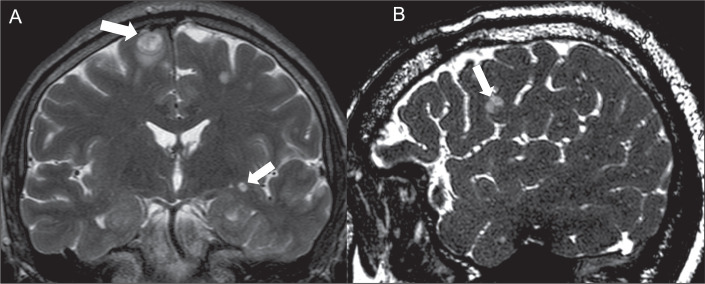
MRI scans of a patient showing cystic lesions with the dot sign.
